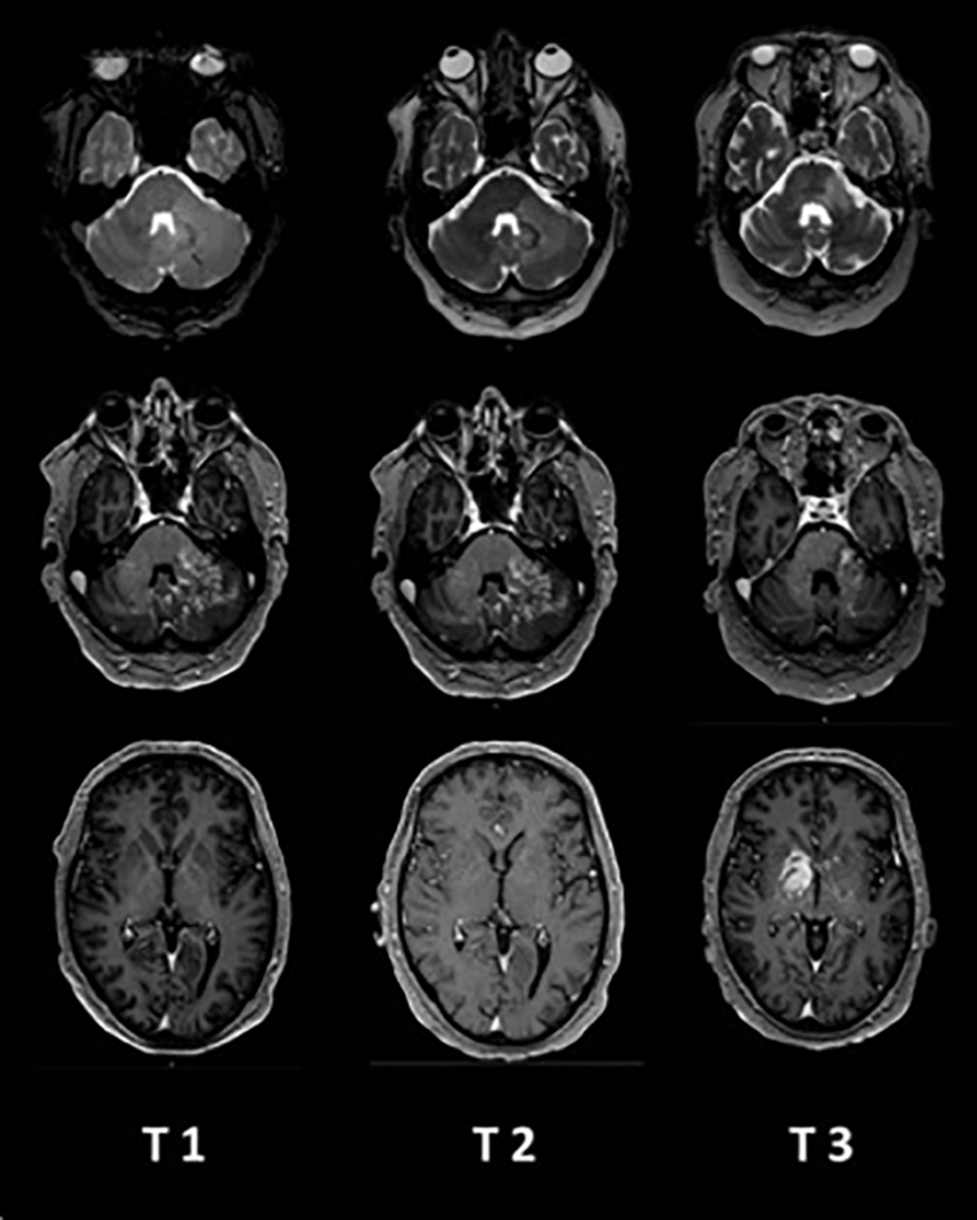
Development of MRI patterns from the time of initial symptoms (T1) to 4 weeks (T2) and 6 months (T3). The upper row shows T2-weighted images of the brainstem, the middle row shows gadolinium enhancement of the brainstem, and the lower row shows gadolinium enhancement of the supratentorial region. MRI, magnetic resonance tomography.
